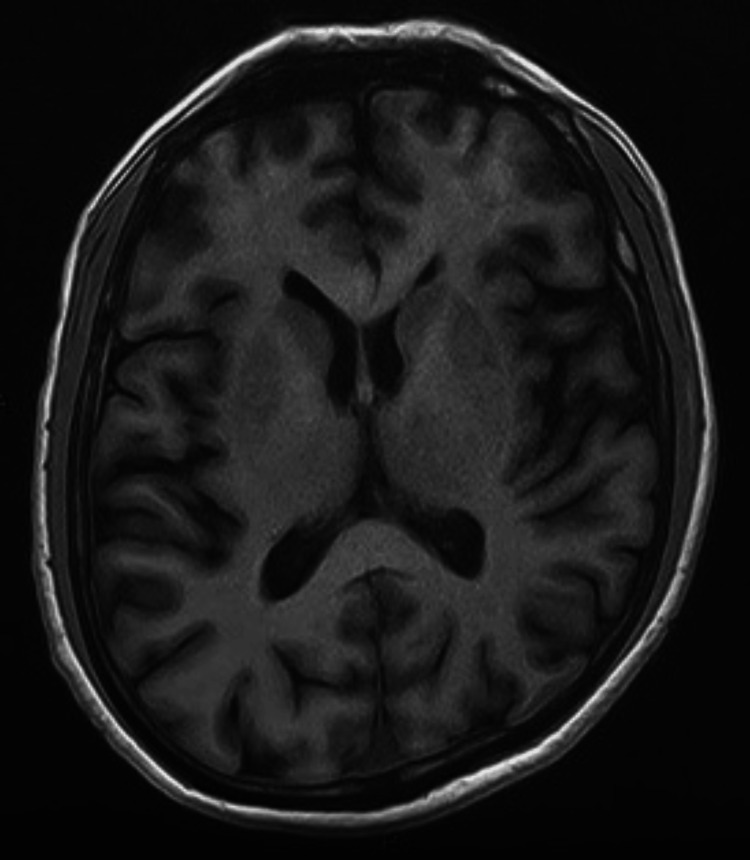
T1-weighted axial MRI plain study of the brain at the level of basal ganglia not showing any abnormality
